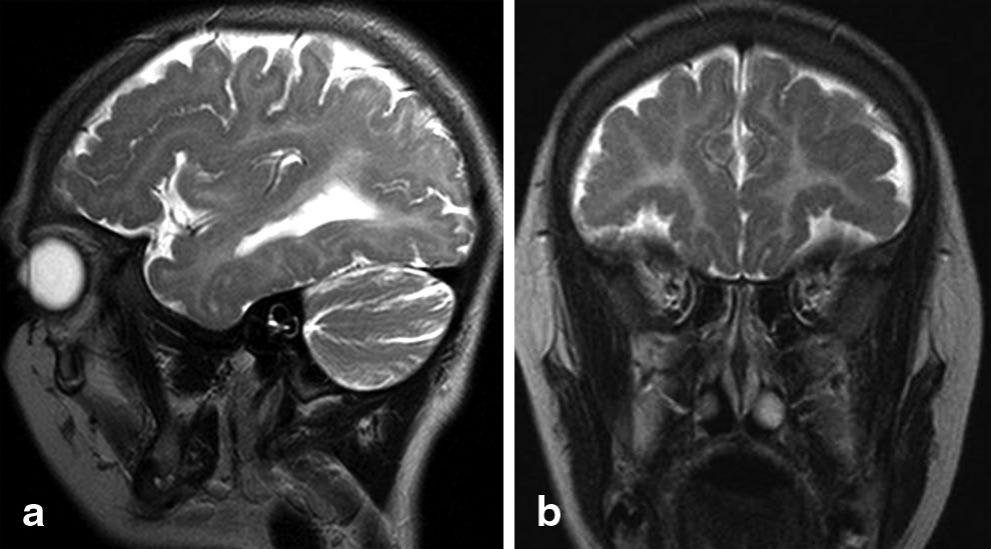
Sagittal (a), and coronal (b) T2-weighted images of case 1 showing irregularity of the frontal cortex with shallow sulci and numerous small gyri, consistent with polymicrogyria
